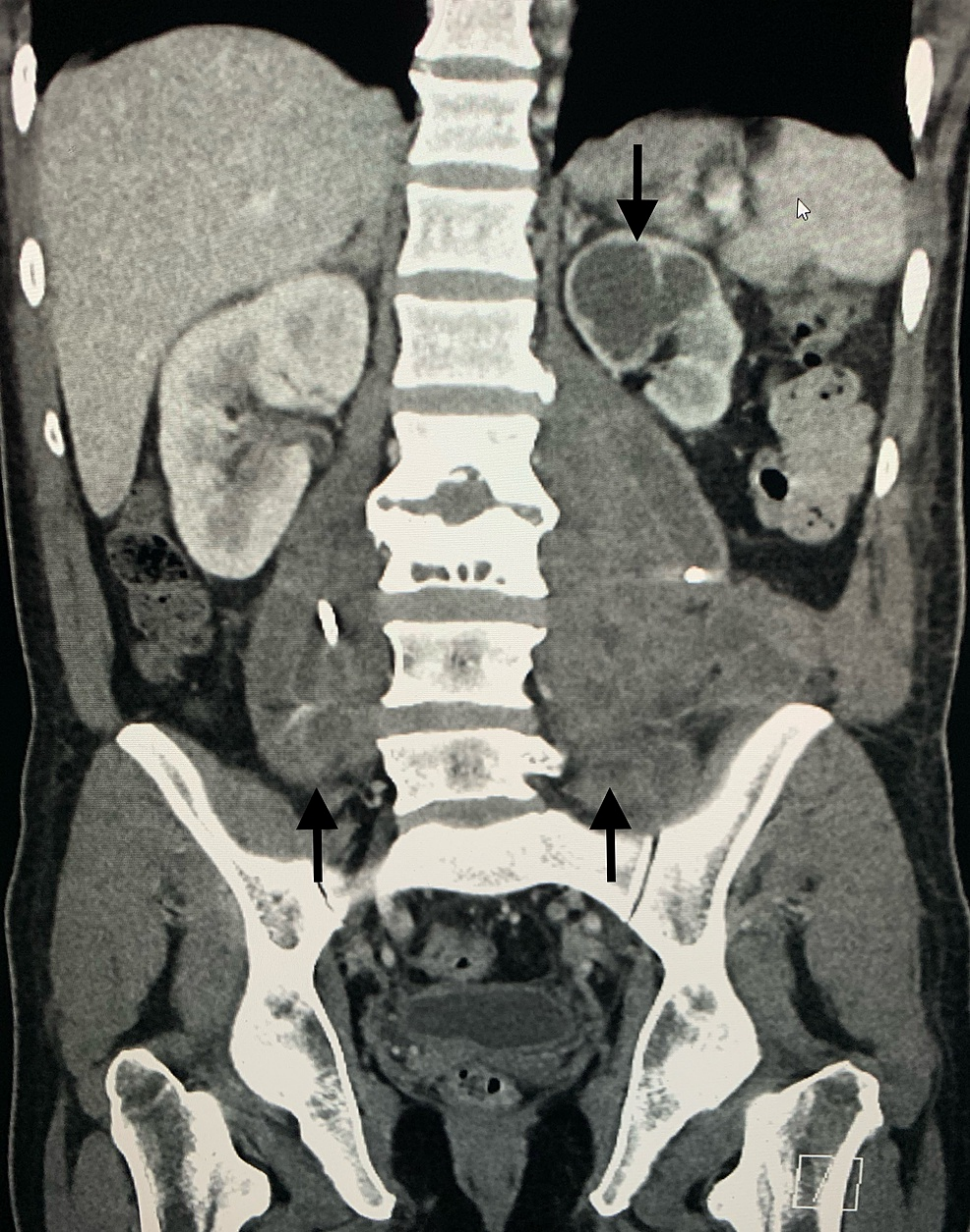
Coronal CT of the abdomen Coronal image of the abdomen at the level of the lumbar spine and kidneys utilizing soft tissue windows. This image demonstrates the extent of the bilateral psoas multiseptated collections. There is also asymmetric atrophy of the left renal superior cortex, a finding commonly seen with chronic renal TB. TB - tuberculosis
